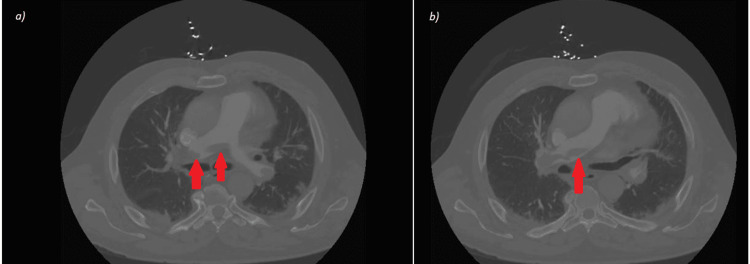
Cross-sectional views of CTA highlighting PE in red arrows. CTA: computed tomography angiography, PE: pulmonary embolism.
